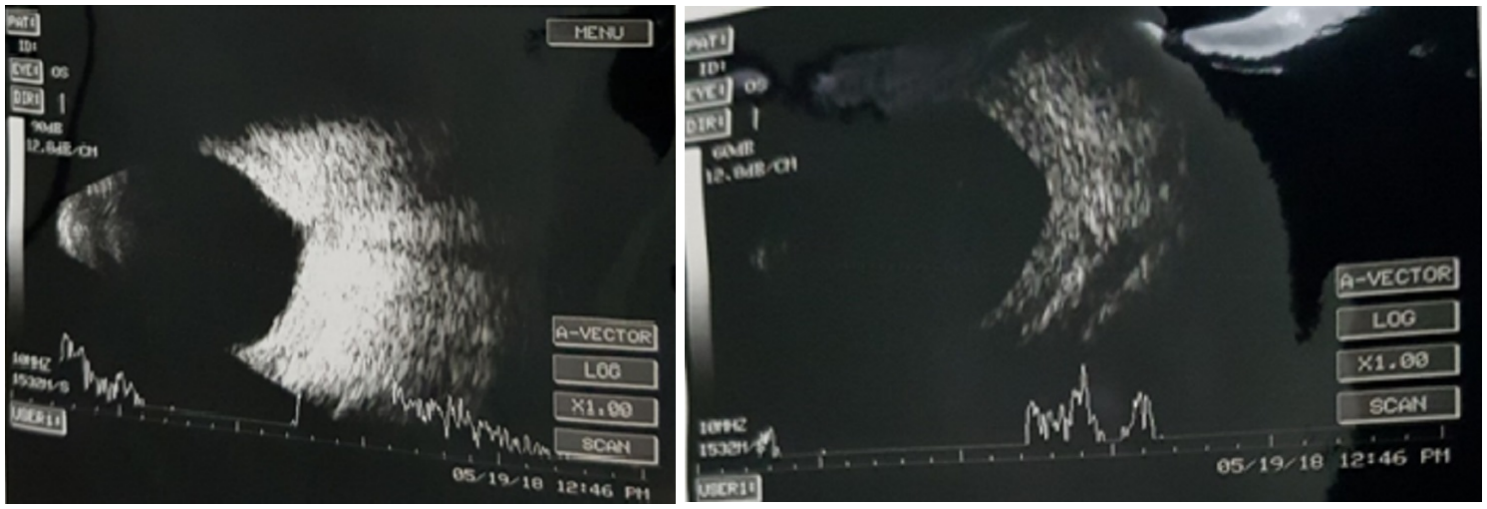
B-scan ultrasonography after 2 weeks in postoperative period showing resolution of choroidal and retinal detachments
